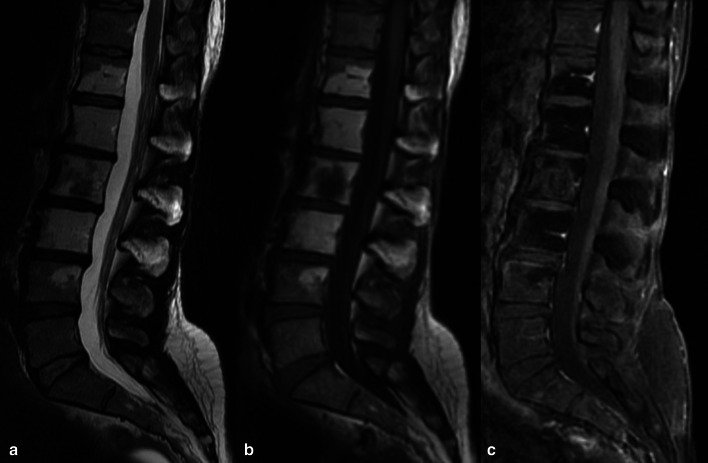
A 46-year-old female patient with history of clear cell renal carcinoma. Sagittal T2-weighted (a), T1-weighted (b) and contrast-enhanced T1-weighted (c) images of the lumbar spine at the level of L2 a bone metastasis previously treated by thermoablation and radiotherapy. Note the bone marrow signal intensity of the vertebral elements in D12-L4 within the irradiation field, due to medullary depletion and fatty replacement
